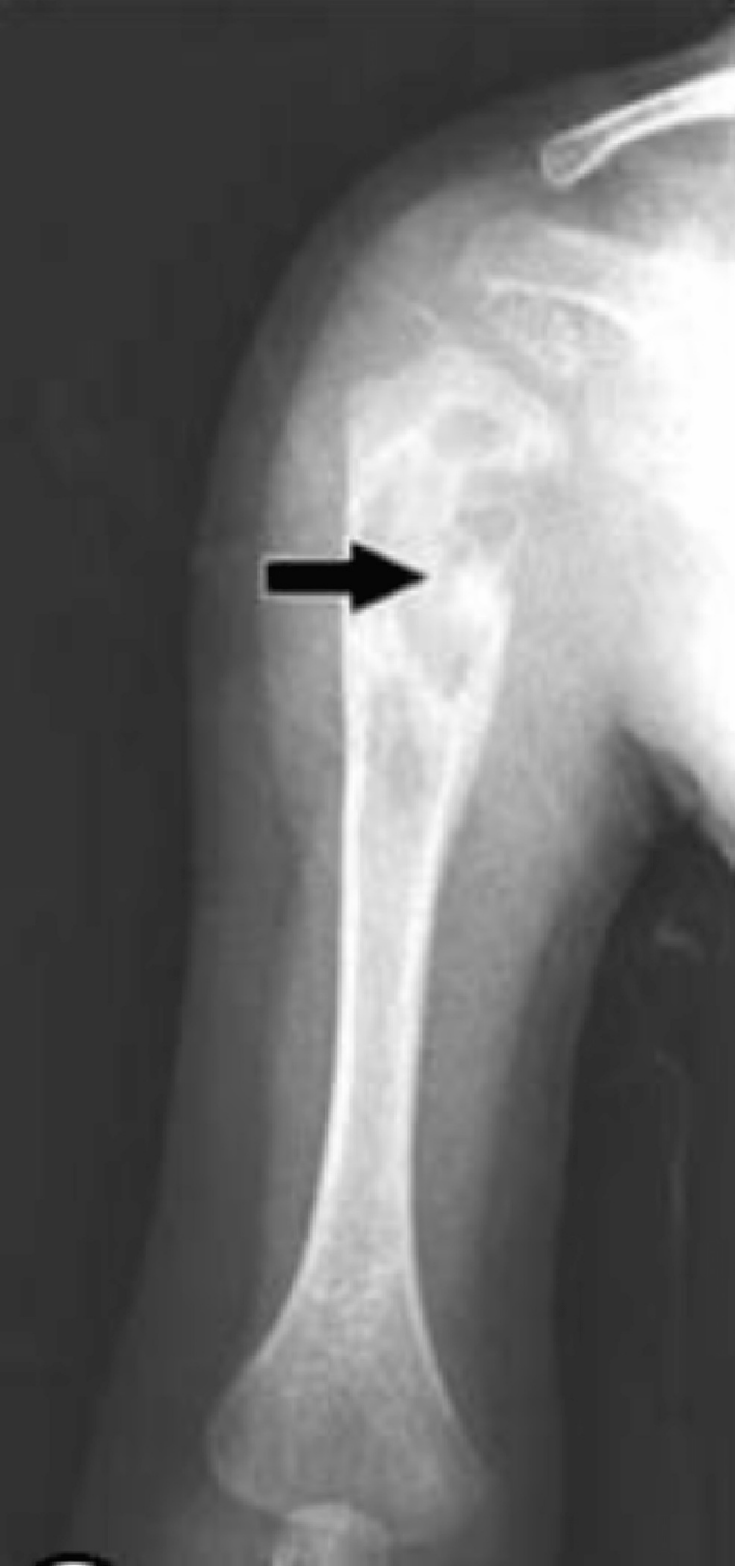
Plain radiograph showing destructive lesion upper metaphysis of the right humerus
